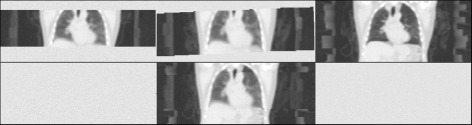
Example patient original and combined-CT images. The registered original CT images from the early (top left), mid (top middle) and late (top right) study sections and the resulting combined-CT for the late section (bottom middle) for an example patient. Grey scale − 1350 < HU < 150
